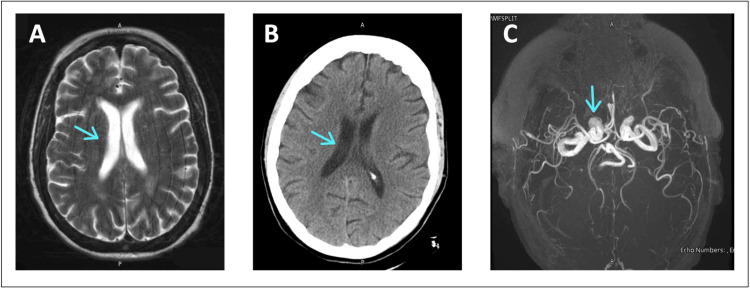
MRI, CT, and MR Angiogram of the Head (A) MRI of the brain without contrast, AX T2 series: mild chronic ischemic changes with no acute intracranial process evident. (B) Axial CT of the head without contrast: no acute intracranial process and no significant interval change. (C) 3D TOF MR angiogram of the circle of Willis without contrast: unremarkable MRA of the circle of Willis. All arrows indicate areas of normal intracranial processes with no acute changes. MRI: magnetic resonance imaging, CT: computed tomography, MRA: magnetic resonance angiography, TOF: time of flight
